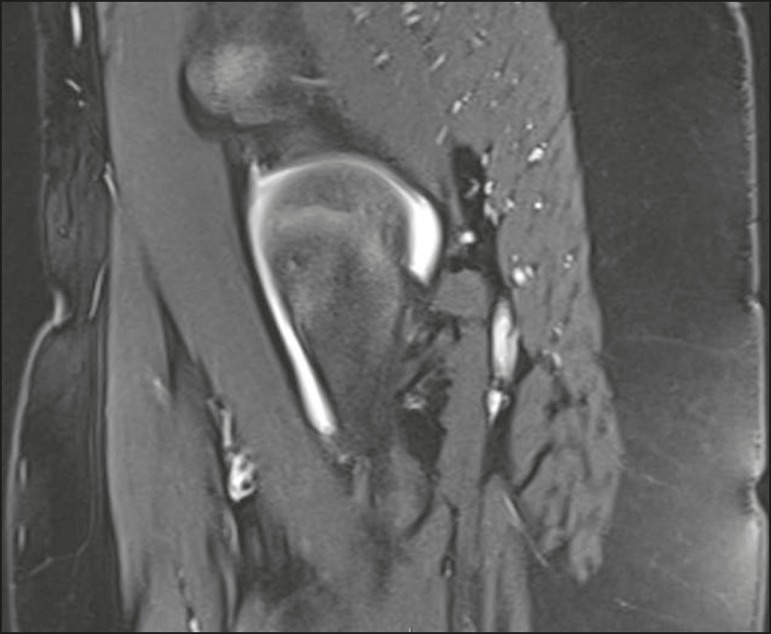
A 12-year-old male patient. Sagittal fat-saturated T2-weighted MRI sequence showing moderate intra-articular effusion in the right hip joint.
